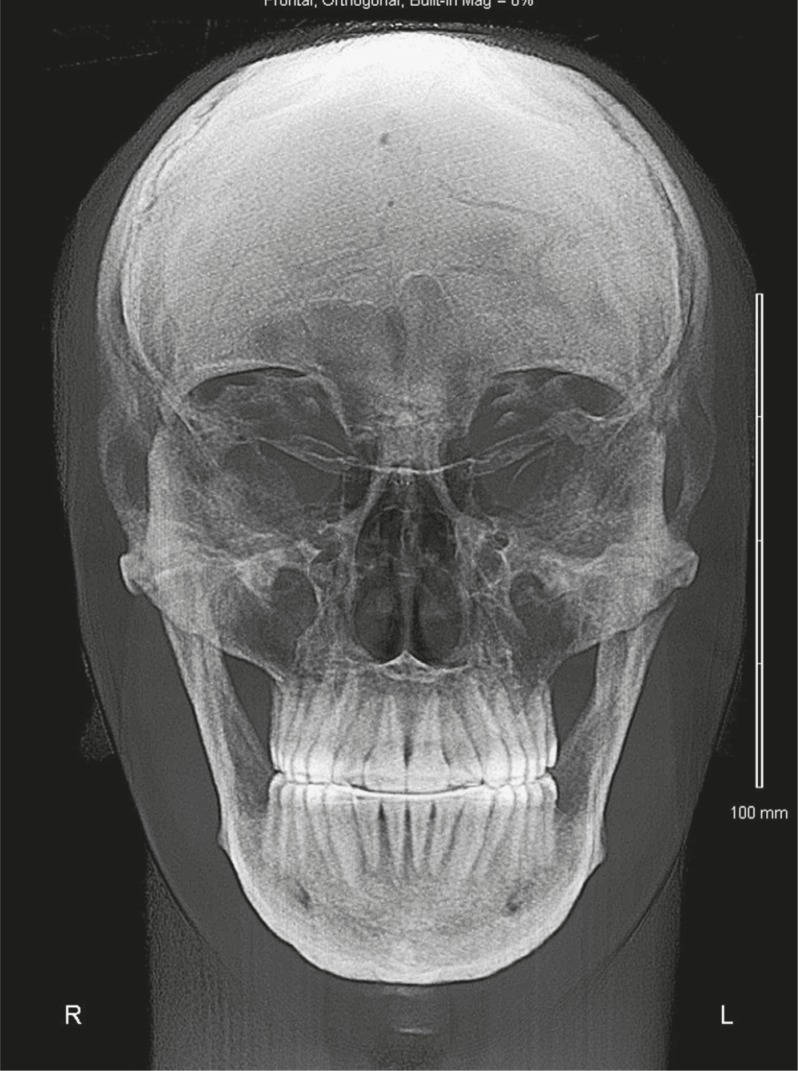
Final frontal cephalometric radiograph
